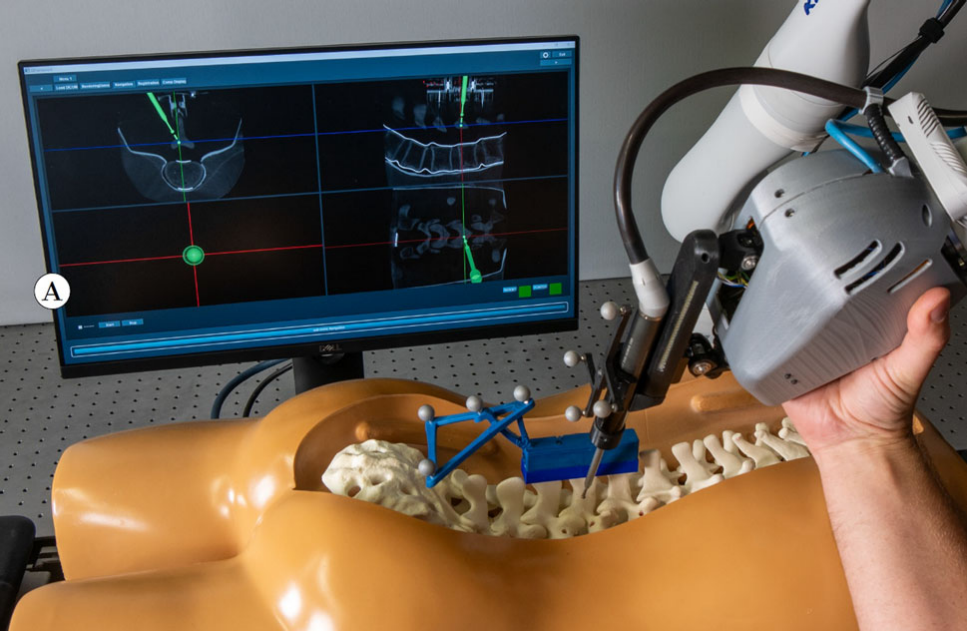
Setup for the prepositioning task: a navigation monitor with a multiplanar view of the CT scan and compensatory display A, the user grasping the tooling robot’s housing, and the torso with a spine model
